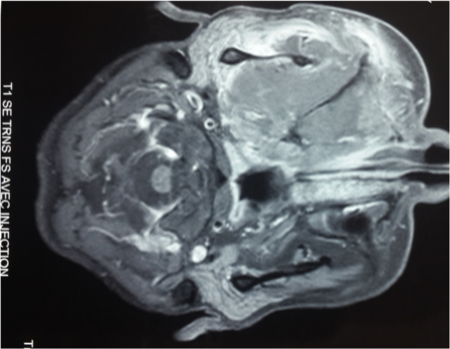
Axial T1-weighted magnetic resonance image of the facial area showing heterogeneous contrast enhancement and necrotic areas
